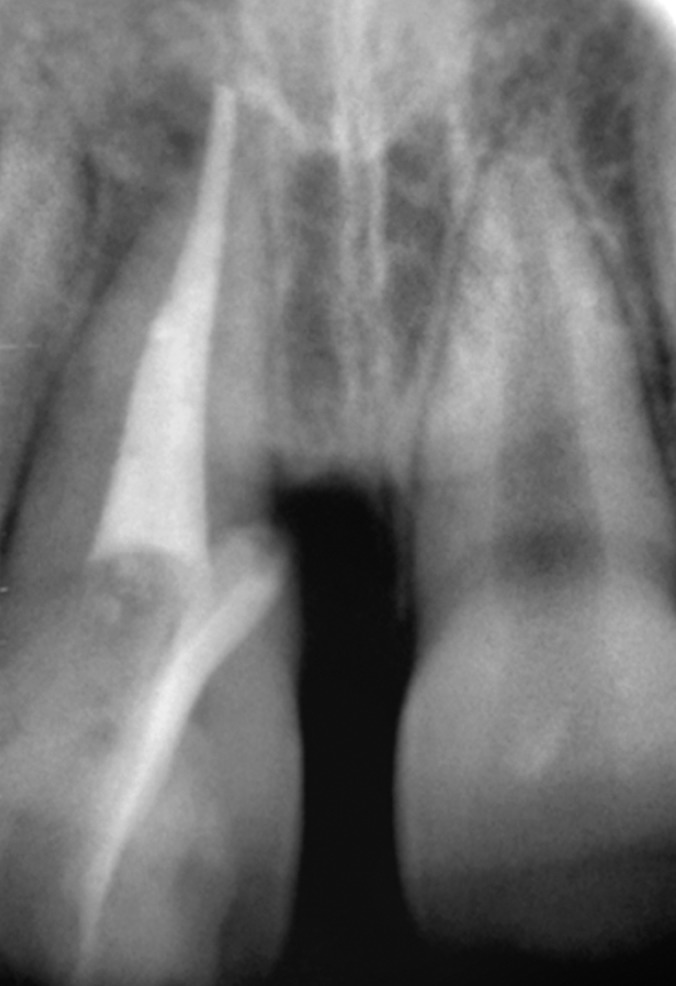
Radiograph of the tooth after splint removal
